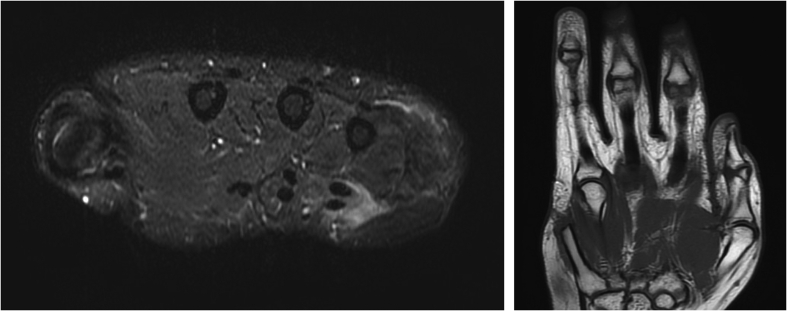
MRI of the right hand and fingers in October 2022 showing the prior small finger ray amputation, with no evidence of disease recurrence.
